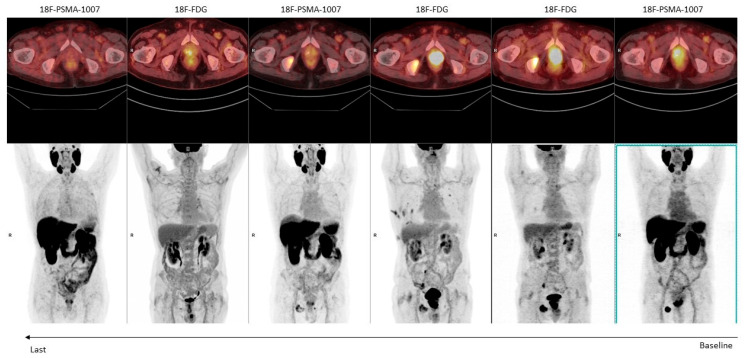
An 82-year-old man with a high-risk prostate cancer (Gleason score: 4 + 5) undergoing hormonal therapy. Serial [^18^F]FDG and [^18^F]-PSMA-1007 PET/CT examinations were performed to monitor the response to treatment (from right to left).
